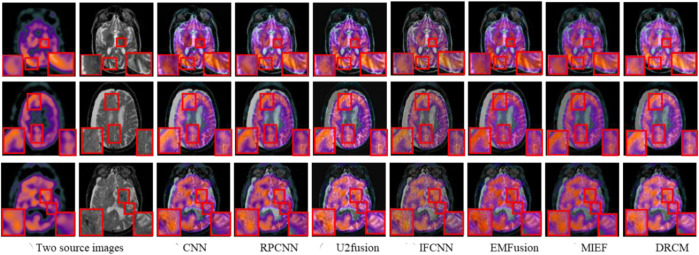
SPECT–MRI fusion images under different comparison methods. Two source images are reproduced with permission from http://www.med.harvard.edu/AANLIB/home.html.
